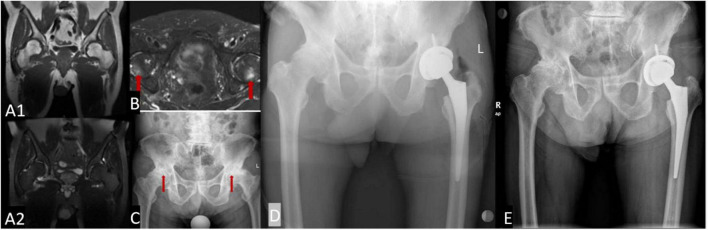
The morphology of the acetabulum and femoral head is bilaterally normal, with uniform internal signals (A1,A2); when underwent 3 months of radiotherapy, femoral head with slightly longer T1 and T2 signals, and uneven and patchy internal signals (B). Bilateral hip joint space narrowing is seen on X-ray examination, and the articular surface is less smooth. The femoral heads on both sides have become flattened and show uneven density (C). Post-operative examination an artificial hip replacement is seen on the left hip, which is in a normal position (D). Two and a half years after surgery, the prosthesis is in normal position (E).
